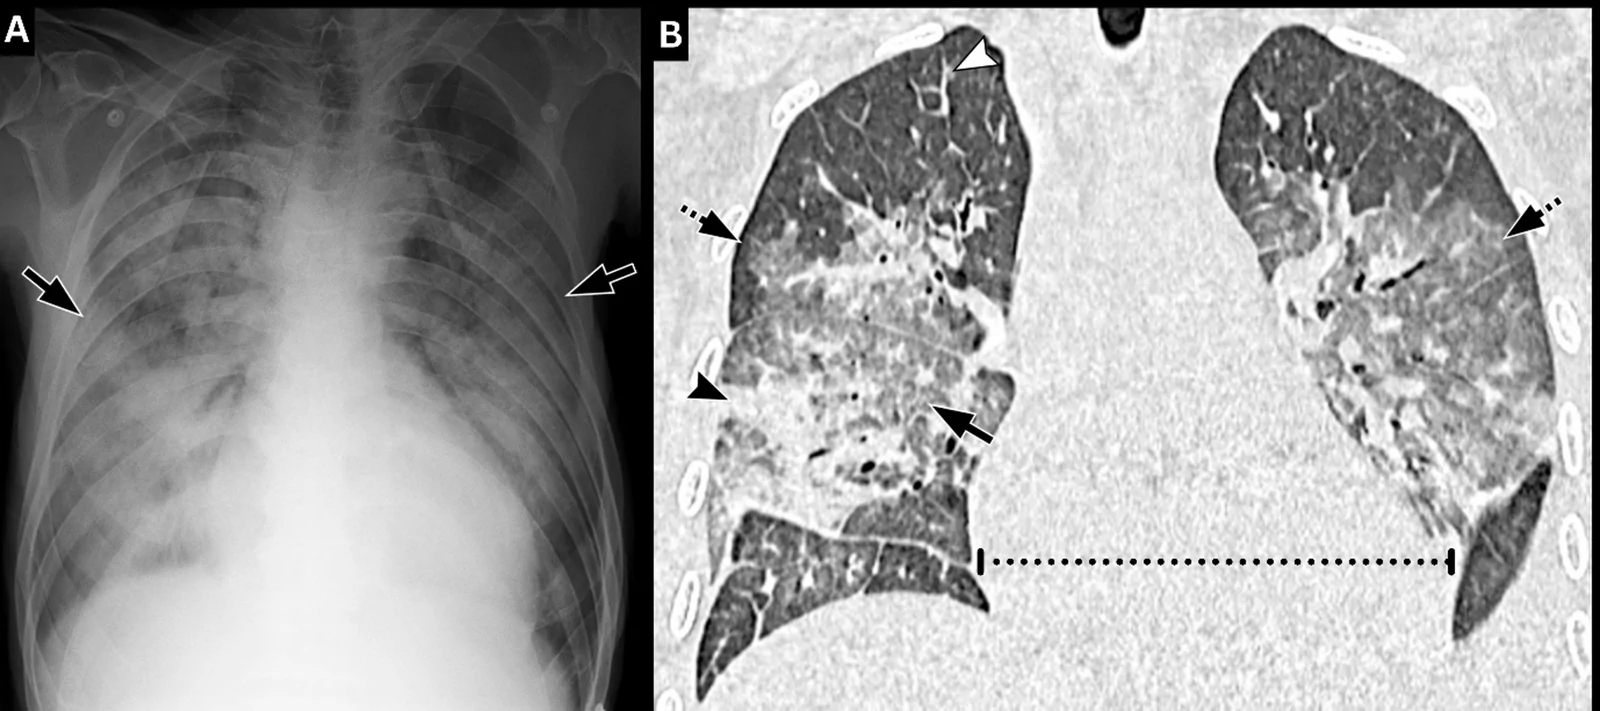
Pulmonary manifestations in scrub typhus. A Chest radiograph in a 70-year-old male with 4 days of fever and 1 day of dyspnea shows diffuse bilateral consolidation (solid black arrows), consistent with pneumonia. He was treated with IV doxycycline for 8 days & discharged 6 days later. B Coronal HRCT chest in a 20-year-old female with fever and dyspnea demonstrates bilateral perihilar ground-glass opacities (dotted black arrows) with patchy consolidation (black arrowhead), smooth interlobular septal thickening (solid black arrow), and cardiomegaly (black dotted line), consistent with interstitial pulmonary edema
